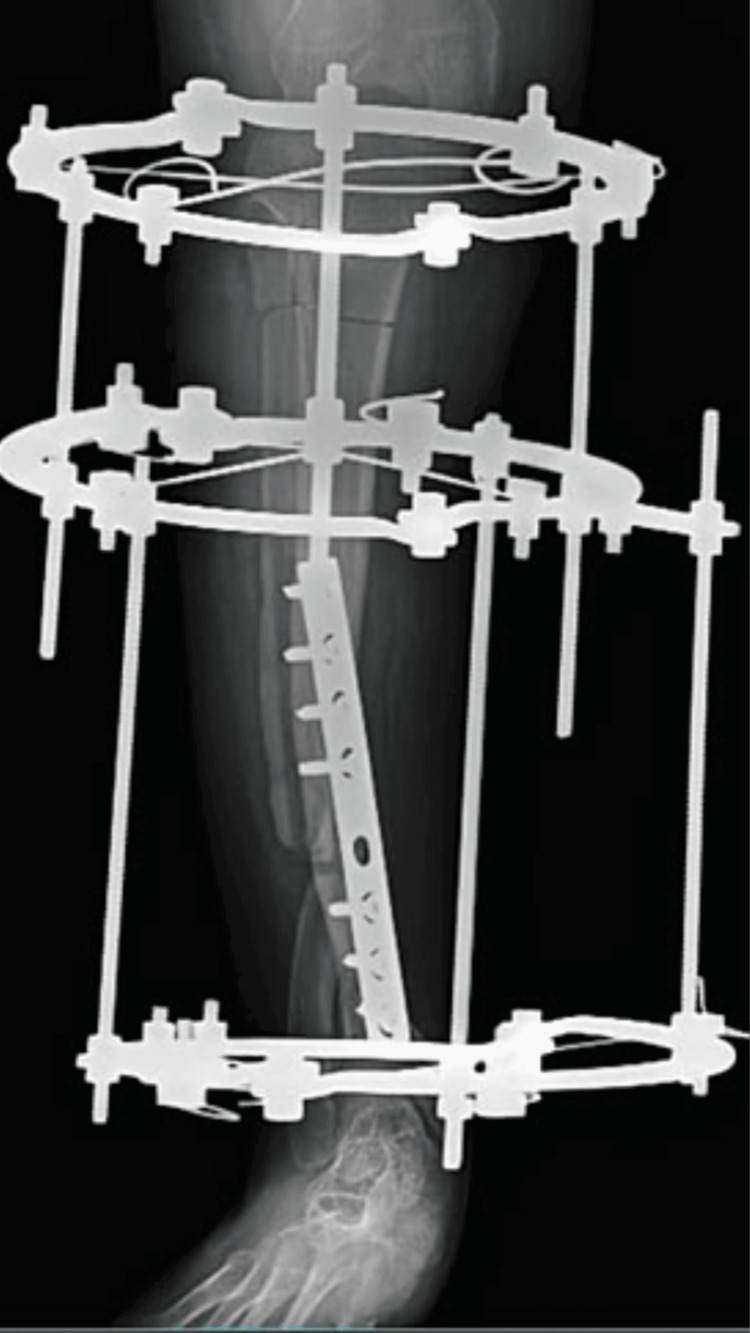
X-ray of right tibia showing Ilizarov external fixator
